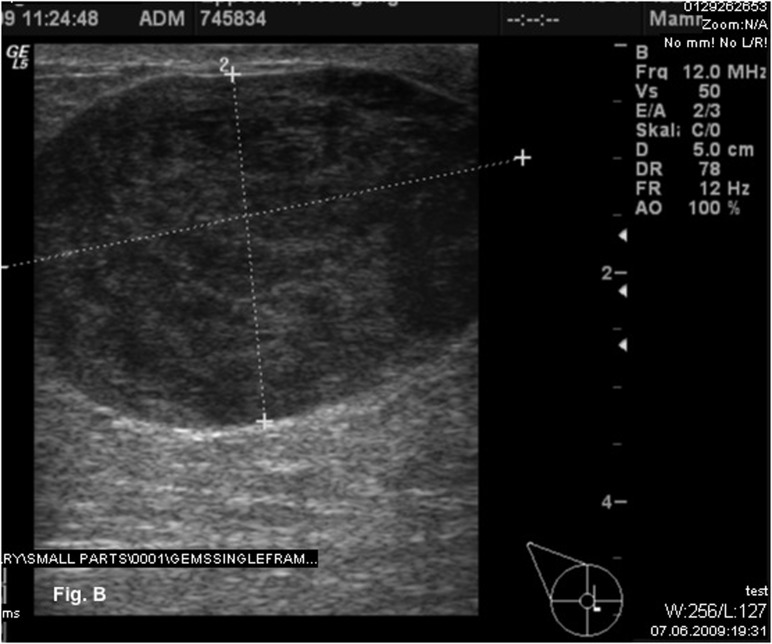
Ultrasonography showed a hypoechoic solid mass suggestive of a fibroadenoma.
